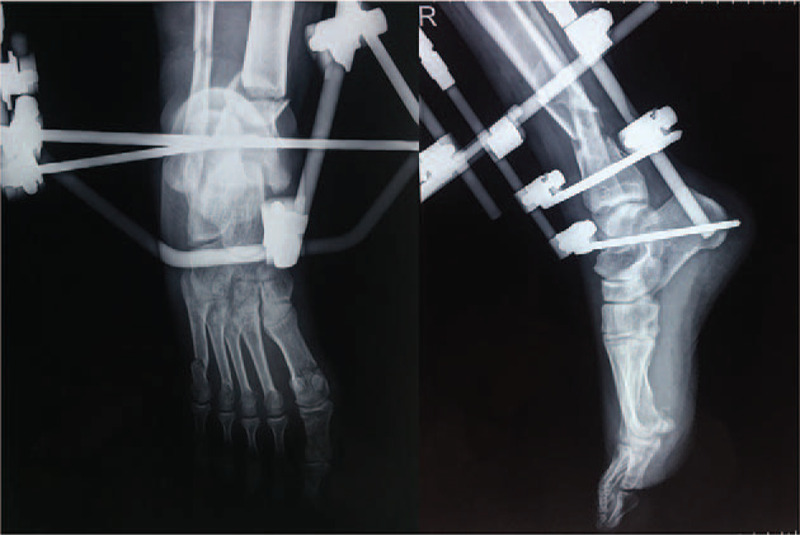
Digital radiograph at day 14 post-injury. Dislocations of the tibia and fibula were partially corrected under traction using an ankle-spanning external fixator.
